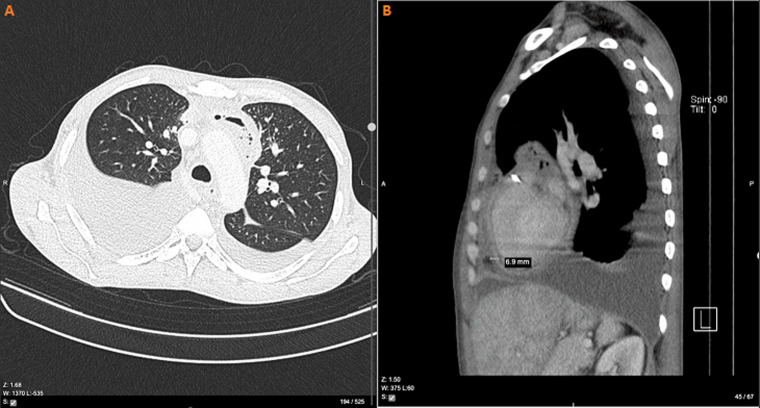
(A, B) CT scan of the chest showing bilateral partial atelectasis, more on the right lung and pericardial thickening of about 6.9 mm
